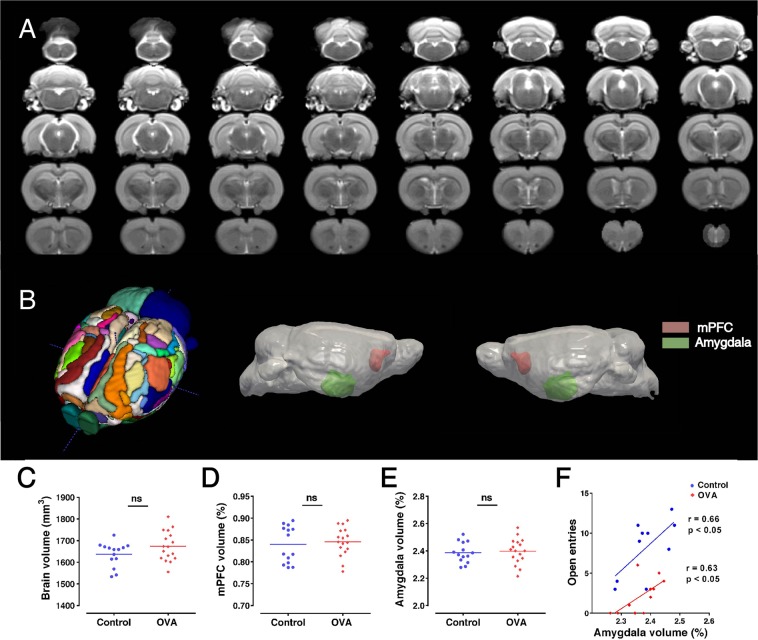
Anxiety-like behavior is negatively correlated with amygdala volume. (A) Representative T2-weighted coronal MRI slices, which were registered to template. (B) Displays three-dimensional rendering views of atlas (left panel), lateral representative of brain (middle and right panel). Regional selections of mPFC and amygdala have shown red and green, respectively. (C–E) MRI volumetric measurements of whole brain, mPFC and amygdala, respectively. The horizontal bars represent mean values (as percent of brain volume). Data were analyzed by t-test (Control: n = 14, OVA: n = 17). (F) The number of entries to the open sections in elevated zero maze (inversely related to anxiety) was positively correlated with amygdala volume. ns, not significant; OVA, ovalbumin; mPFC, medical prefrontal cortex.
